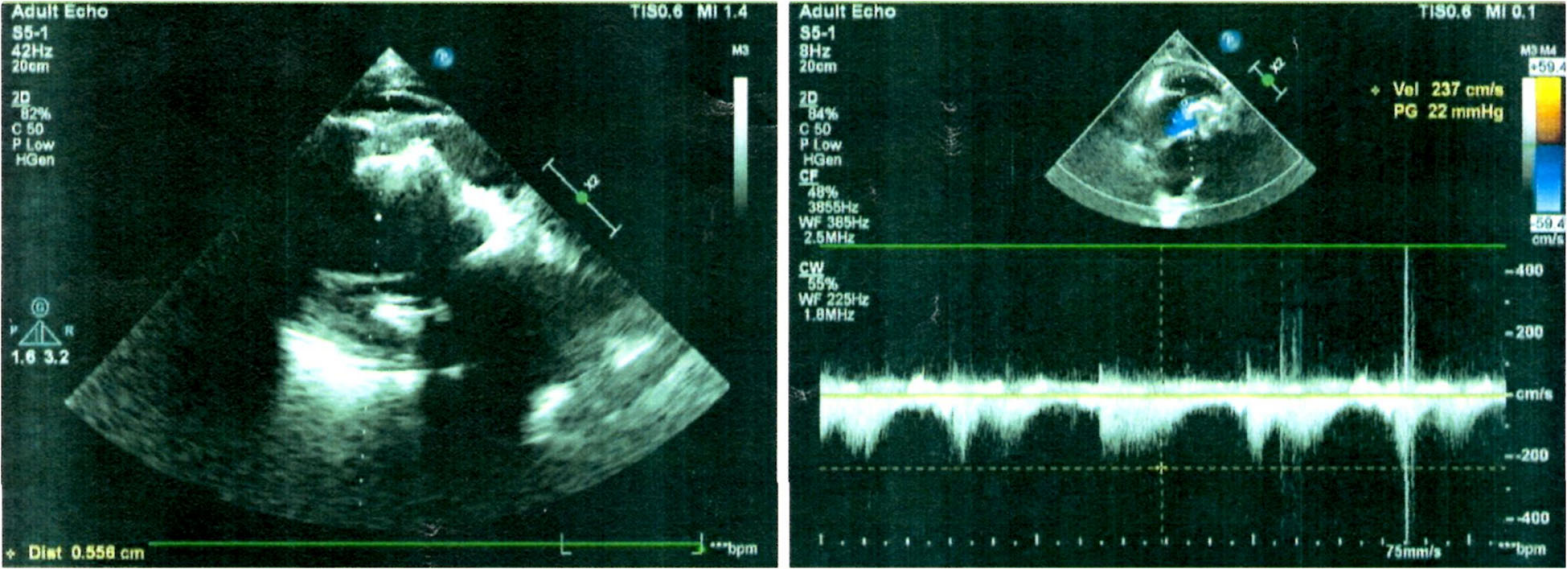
Echocardiography shows extensive endocardial calcification of the left ventricle
